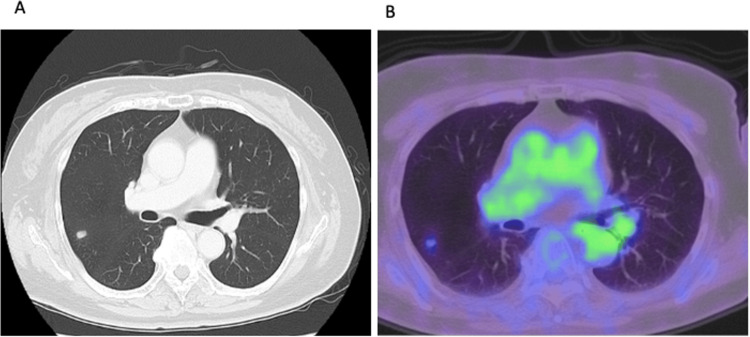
Pulmonary nodule detected during pembrolizumab therapy. (A) Computed tomography (CT) demonstrating a newly developed pulmonary nodule in the right lung. (B) Positron emission tomography (PET) showing mild fluorodeoxyglucose uptake in the right upper lobe lesion without uptake in previously involved lymph nodes.
